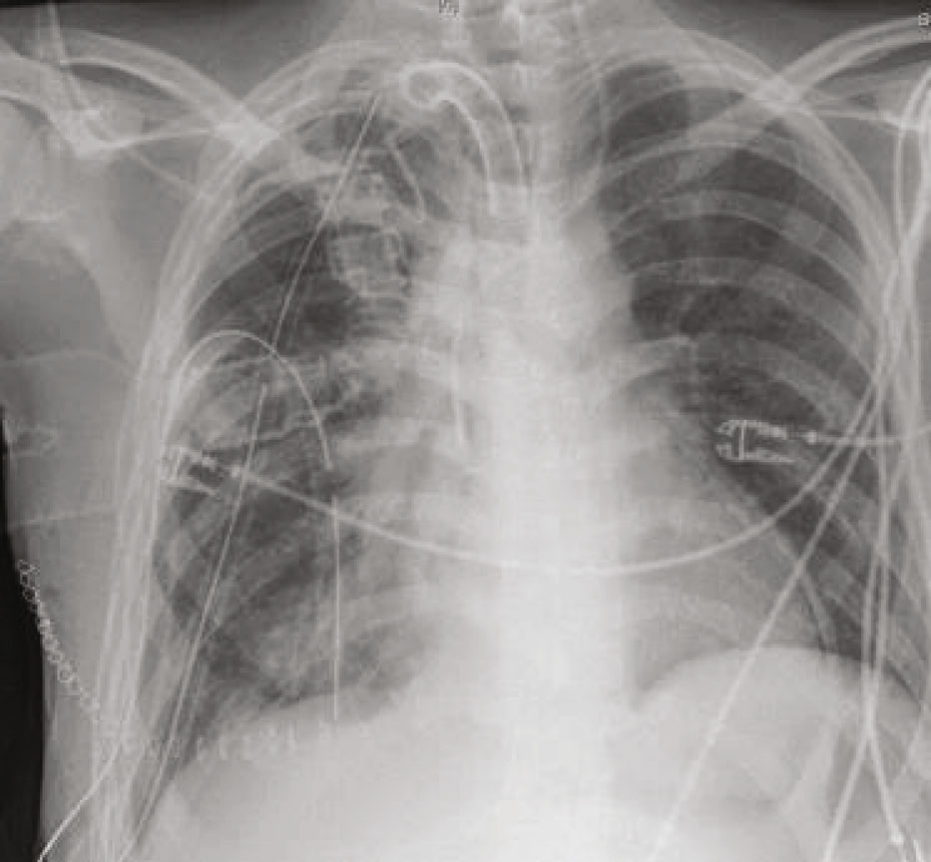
Chest X-ray after the transplantation.
